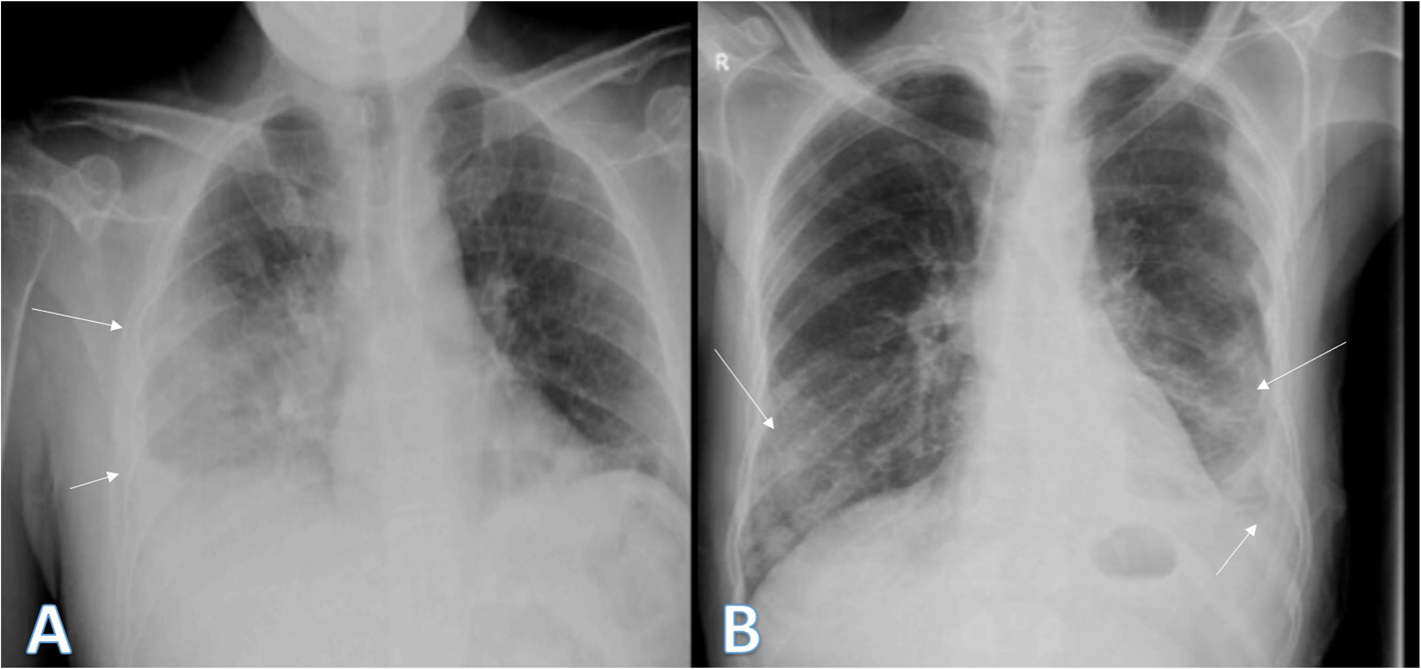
Two different patients with positive COVID-19. a 34-year male was complaining of cough and fever. Follow-up chest X-ray showed mild right pleural effusion (short arrow) with right mid and lower zonal air space consolidation opacities (long arrow). The total severity score was 2. b 61-year male was complaining of fever, cough, and diarrhea. Follow-up chest X-ray showed mild left pleural effusion with bilateral lower zonal air space consolidation opacities (long arrows). The severity score was 2 for each lung, so TSS was 4
